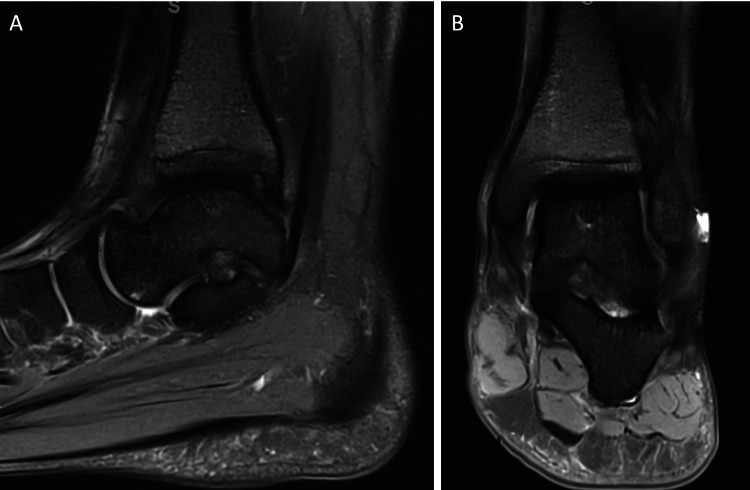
MRI of the left ankle at 2.5 years from injury - April 15, 2023. (A-B) T2-sequence, sagittal, and coronal views show significant improvement of the talar osteonecrosis with no “double line sign” and almost complete resolution. The posterior tibial plafond re-demonstrates a stable small osteochondral defect.
